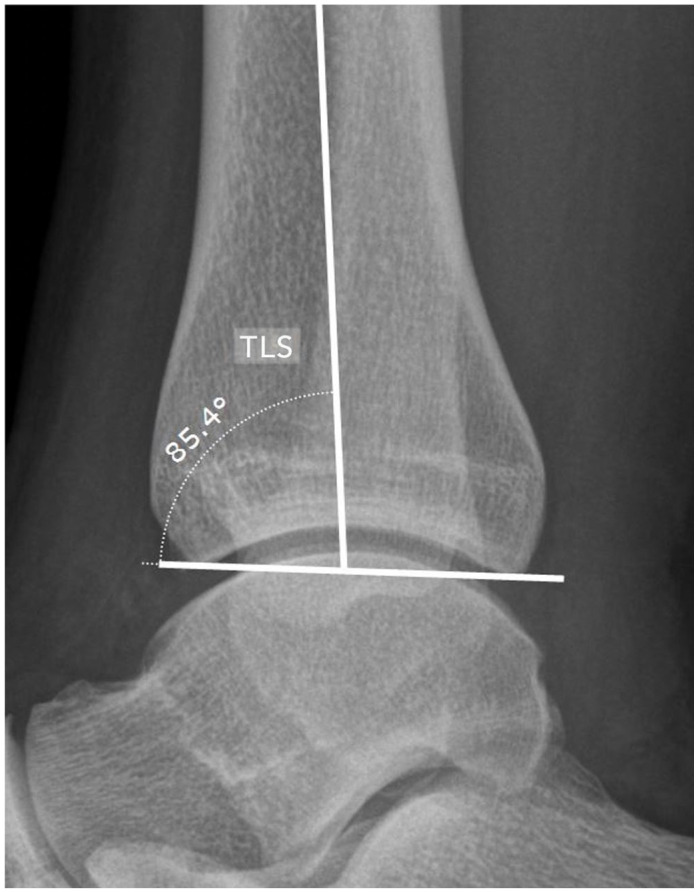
Measurements of the tibial lateral surface (TLS) angle on a lateral weightbearing radiograph: The TLS angle is the angle between the tibial shaft axis and a line extending from the anterior border to the posterior border of the distal tibia, measured in degrees.
